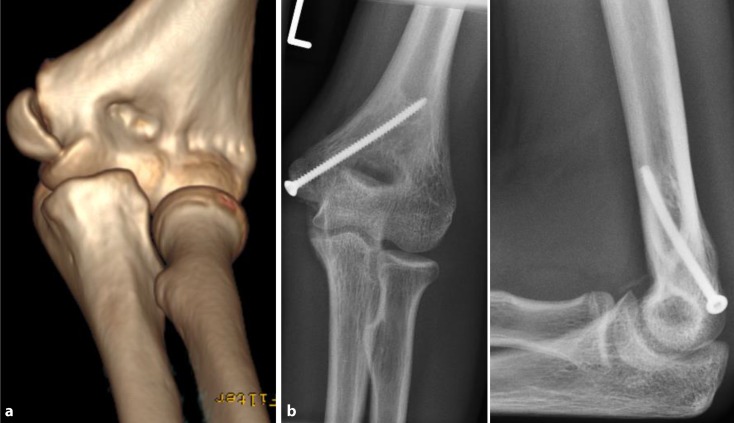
Extra-articular avulsion of the ulnar epicondyle, AO 13 A1.2 (14-year-old female patient). a Preoperative computed tomography (CT), b owing to the small fragment, the fracture is treated with only one screw
